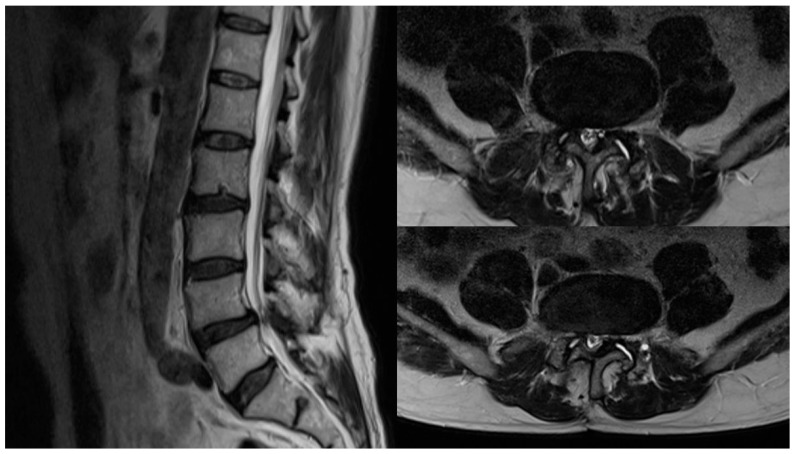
Sagittal and axial lumbar MRI images of the patient in the preoperative period [Axial images demonstrate the L4–L5 intervertebral disc space].
